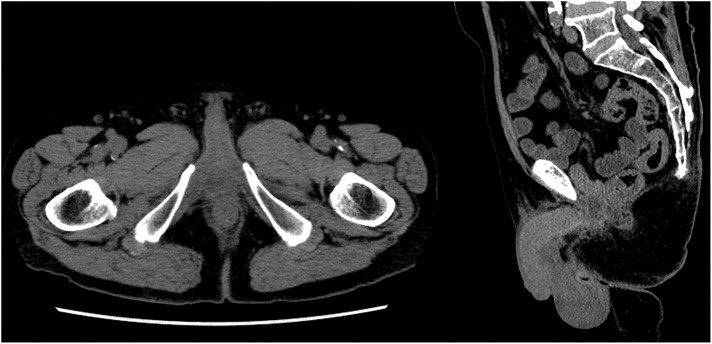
CT at 6 months postoperatively showing no evidence of hernia recurrence.
